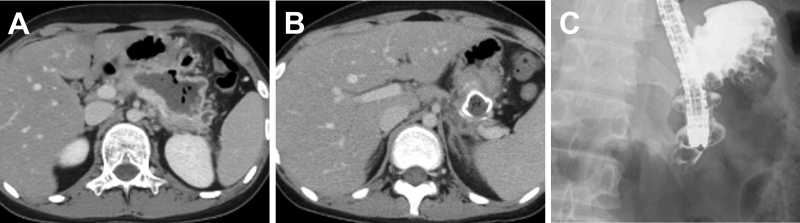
Resolution of the WON after pancreatic duct decompression. (A) CT before pancreatic duct stenting showing a persistent WON. (B) CT after stenting demonstrating marked shrinkage of the WON without further necrosectomy. (C) Subsequent contrast study showing complete disappearance of the communication between the WON and the main pancreatic duct, indicating resolution of DPDS. CT, computed tomography; DPDS, disconnected pancreatic duct syndrome; WON, walled-off necrosis.
